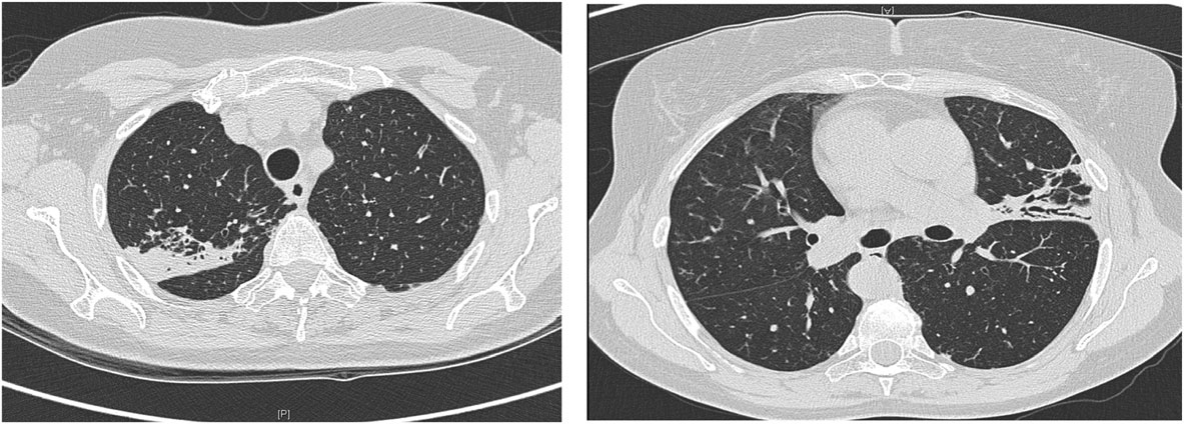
CT Thorax 2007: CT Thorax from 2007 demonstrating bi-apical scarring, right middle lobe atelectasis, right upper lobe cylindrical bronchiectasis and reticulo-nodular densities in both lower lobes
